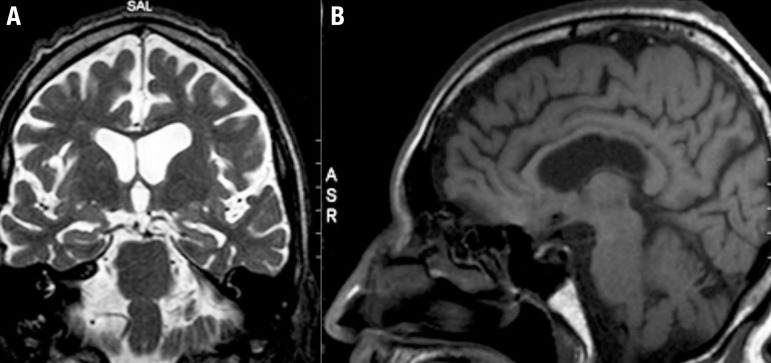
Brain MRI showing: [A] (T2-weighted coronal section) mild hippocampal atrophy; [B] (sagittal T1 section) demonstrates: mild cerebellar and posterior callosum atrophy.
